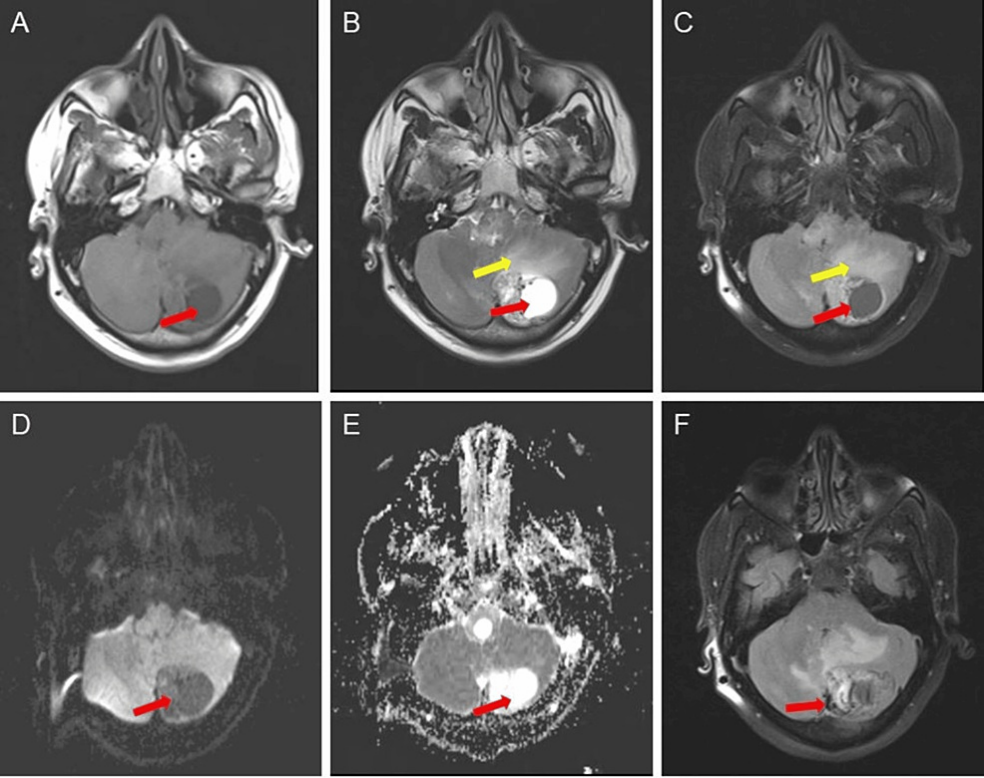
Multi-sequence MRI findings of a lesion in the left cerebellar hemisphere, demonstrating varied signal characteristics and associated features A) Axial T1 MRI image showing a hypointense solid-cystic lesion in the left cerebellar hemisphere (red arrow); B) T2 axial MRI image showing a hyperintense cystic component (red arrow) with T2 hyperintense vasogenic edema (yellow arrow); C) FLAIR axial image showing a hypointense lesion in the left cerebellar hemisphere (red arrow) with surrounding FLAIR hyperintense vasogenic edema (yellow arrow); D) DWI image showing no areas of diffusion restriction in the lesion; E) ADC map showing a bright signal in the lesion (red arrow); F) GRE sequence showing a focus of blooming suggesting hemorrhage or calcification (red arrow) FLAIR: Fluid attenuated inversion recovery; DWI: Diffusion-weighted imaging; ADC: Apparent diffusion coefficient; GRE: Gradient recalled echo
